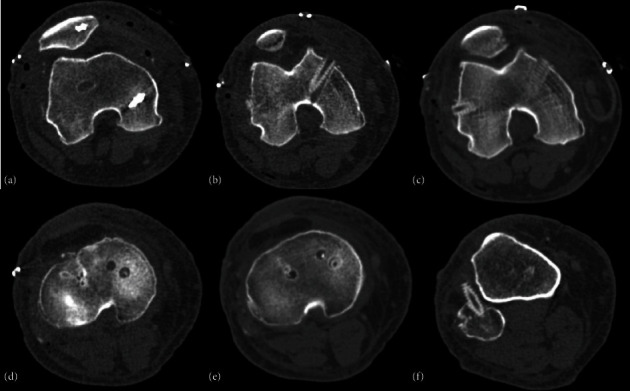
CT scan following multiligamentous reconstruction demonstrates adequate reduction of dislocation. (a–c) tunnel trajectory is identified as coursing caudocephalically toward the lateral femoral condyle, with an oblique disposition. Additionally, there is discreetly oblique cephalocaudal tunneling of the medial femoral condyle, reaching the inner portion of the said condyle. (d, e) Oblique tunneling of the internal tibial plateau is noted, with bone fragments within; slightly medial to this trajectory, a second tunnel is partially occupied by a screw. (f) At the level of the proximal epiphysis of the fibula, complete anteroposterior tunneling is identified, partially occupied by a fixation screw.
